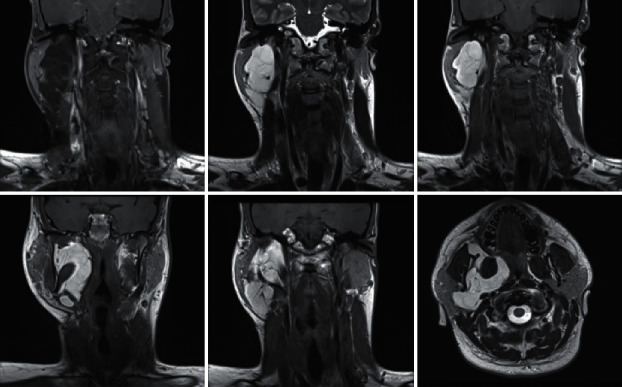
MRI of the neck reveals an enhancing homogeneous lesion seen to extend from level of the base of skull surrounding the lateral pterygoid muscle down to the pharyngeal space down to the lower neck level of the cricoid cartilage. Mass appears to measure 10.0 × 4.8 × 3.5 cm of height, AP, and transverse diameters respectively.
